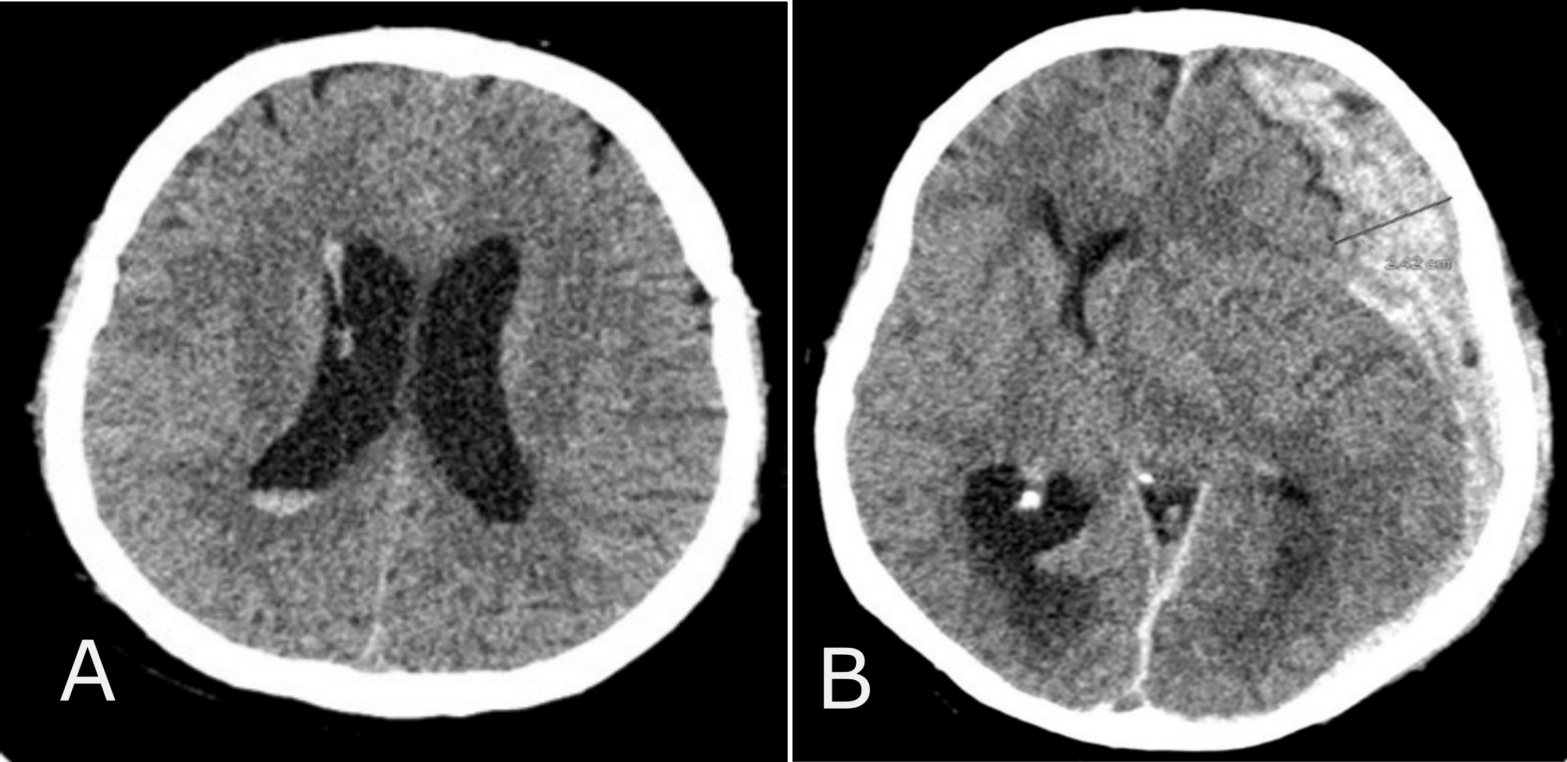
Epidural mass lesion CT findings Epidural space normal findings (A); epidural mass lesion acute epidural hematoma (B).
